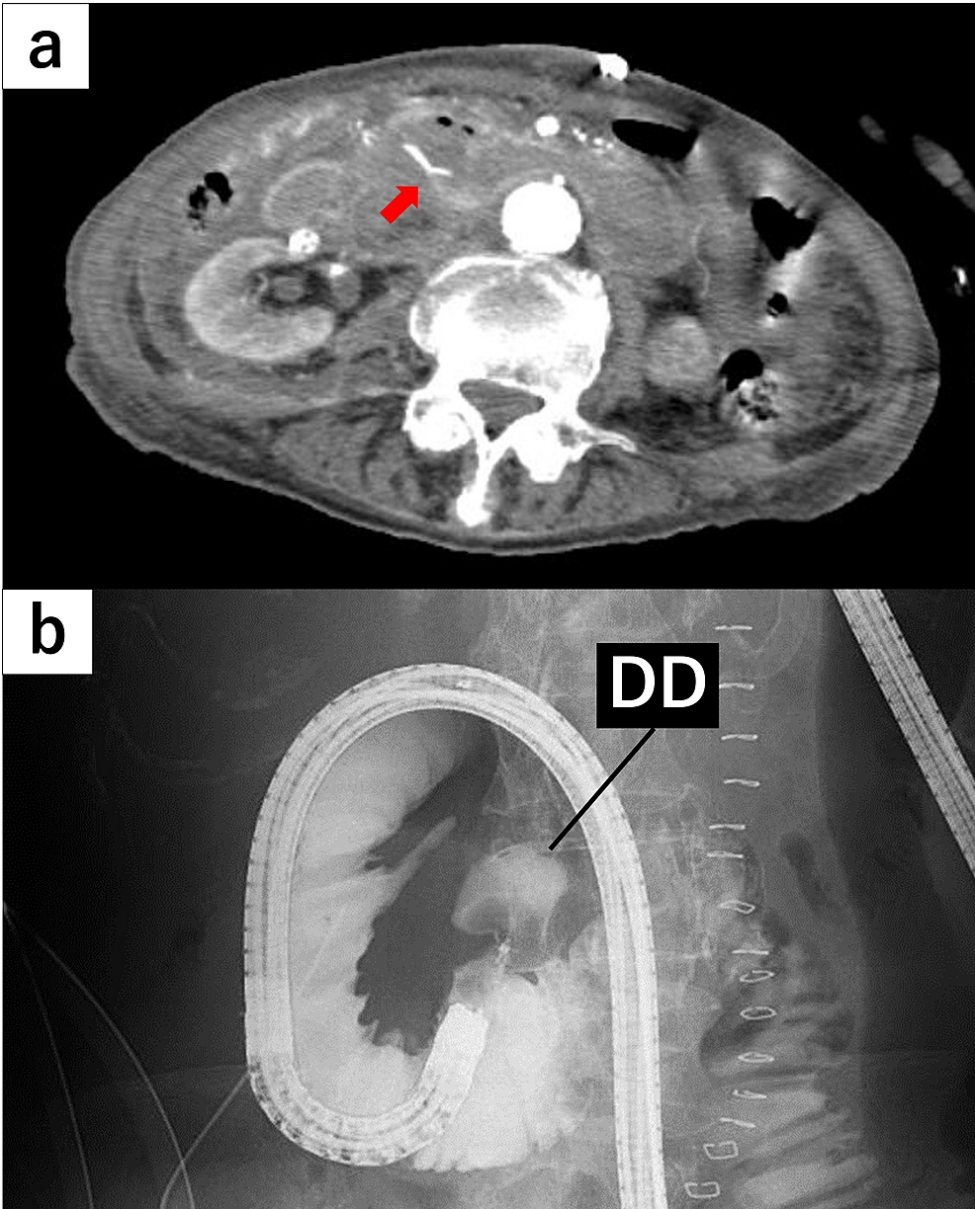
Computed tomography and duodenography. (a) CT scan showing extravasation (red arrow) from the neck of the duodenal diverticulum. (b) Duodenography showing the diverticulum with a small neck arising from the third portion of the duodenum. DD: duodenal diverticulum; CT: computed tomography
